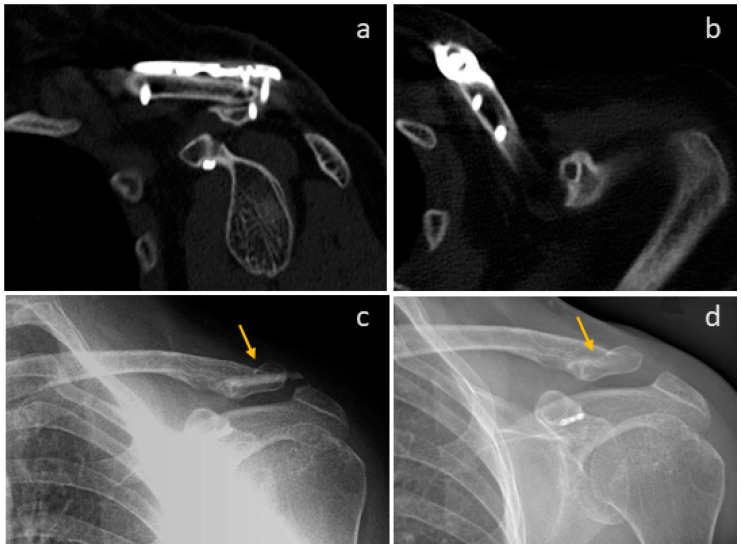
The revision of the non-union of a clavicula of a patient. (a,b): CT-scans: The former metal hardware was removed in the same session. (c): Two Shark Screws^®^ (orange arrows) were used for revision. 12 weeks after revision, bone union was recorded. (d): After 15 months, the Shark Screws^®^ are totally remodelled into the host bone.
